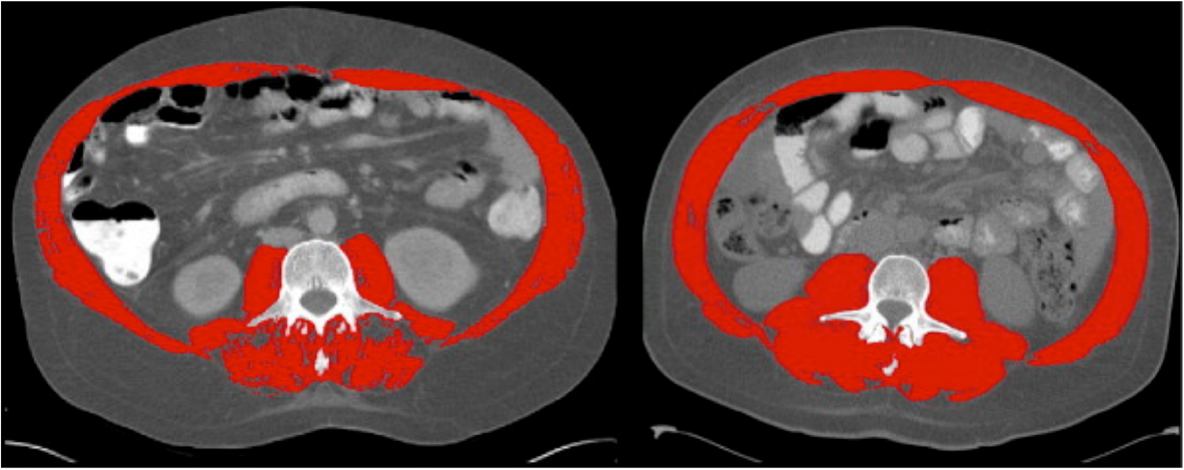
Comparison of sarcopenic and non-sarcopenic computed tomography images at the third lumbar vertebral level. A comparison of two cirrhotic patients with identical BMI (32 kg/m^2^). *Red color* indicates skeletal muscle: rectus abdominis, oblique and lateral abdominal muscles, psoas, and paraspinal muscles. The patient on the *left* is sarcopenic with L3mi of 49.82 cm^2^/m^2^; the patient on the *right* is not sarcopenic with L3mi of 70.8 cm^2^/m^2^. In their study of 112 cirrhotic patients, Montano-Loza et al. used abdominal CT images taken at the third lumbar vertebrae and cutoffs provided by Baumgartner et al. in 1998
